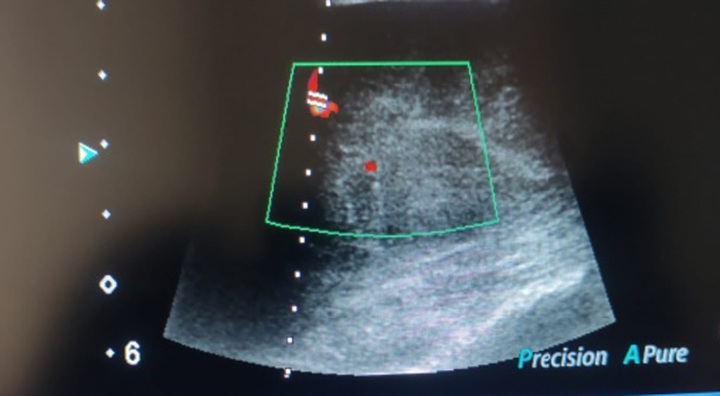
Venous flow within it on colour Doppler imaging.
